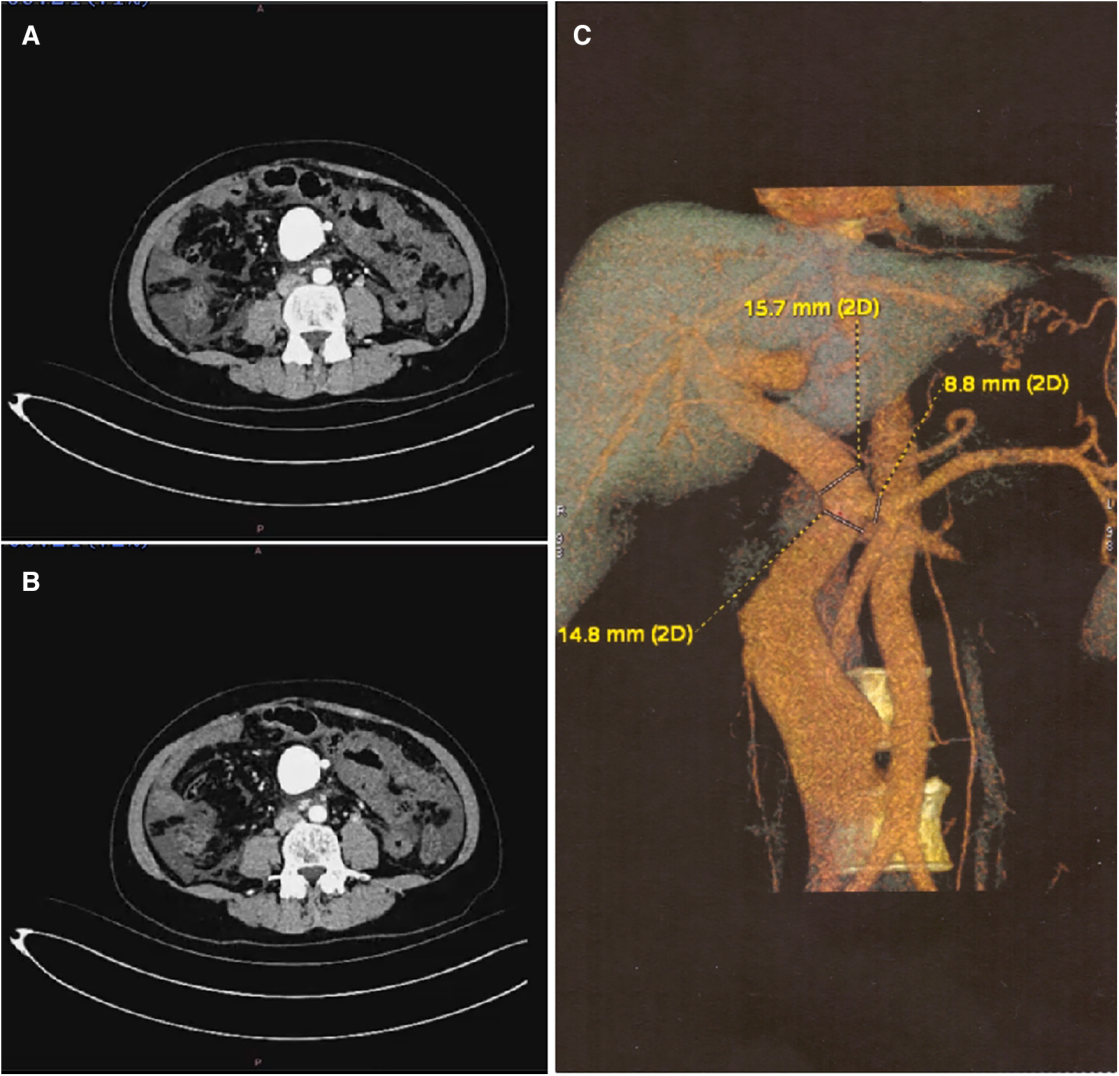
Preoperative Imaging Examination (A,B) CT imaging shows the superior mesenteric artery, fistula, and dilated superior mesenteric vein. (C) Three-Dimensional CT imaging shows the superior mesenteric artery, fistula, and dilated superior mesenteric vein.
